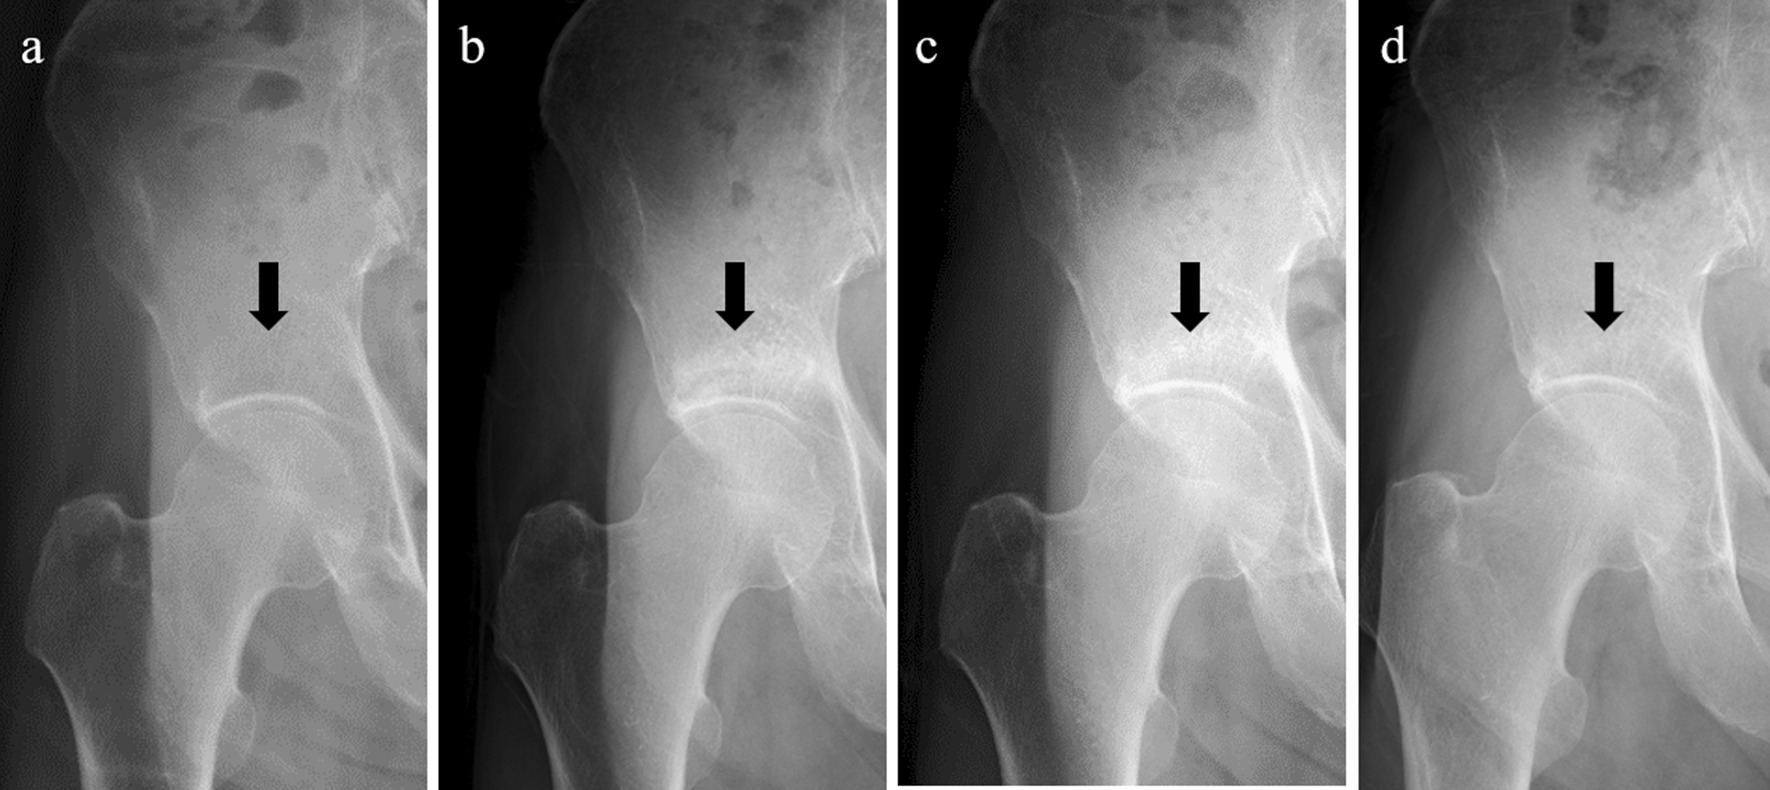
a X-rays at first visit showed no obvious abnormalities. b X-ray at 4 months after the first visit sowed sclerosis of the right supra-acetabulum. c X-ray at 1 year after the first visit. d X-ray at 2 years after the first visit showed the shadow of the sclerosis faded. The arrows show the site of the lesion
